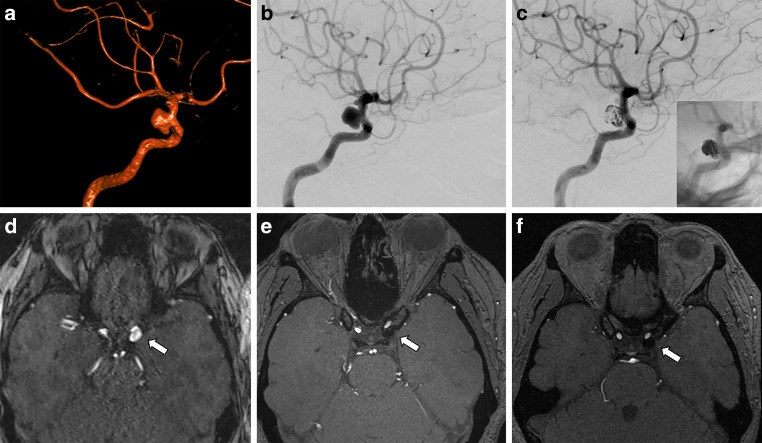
a 3D-angiography showing a saccular multilobulated aneurysm of the left internal carotid artery. b Subtracted digital subtraction angiography (DSA) before endovascular treatment. c Subtracted and unsubtracted DSA after coiling and FRED implantation showing residual aneurysm filling. d Magnetic resonance imaging (MRI) time of flight angiography (TOF) angiography before treatment with an arrow pointing to the aneurysms. e 1-year follow-up MRI TOF-angiography showing punctate residual perfusion of the aneurysm neck. f 6-year follow-up MRI TOF-angiography showing stable complete occlusion of the aneurysm
